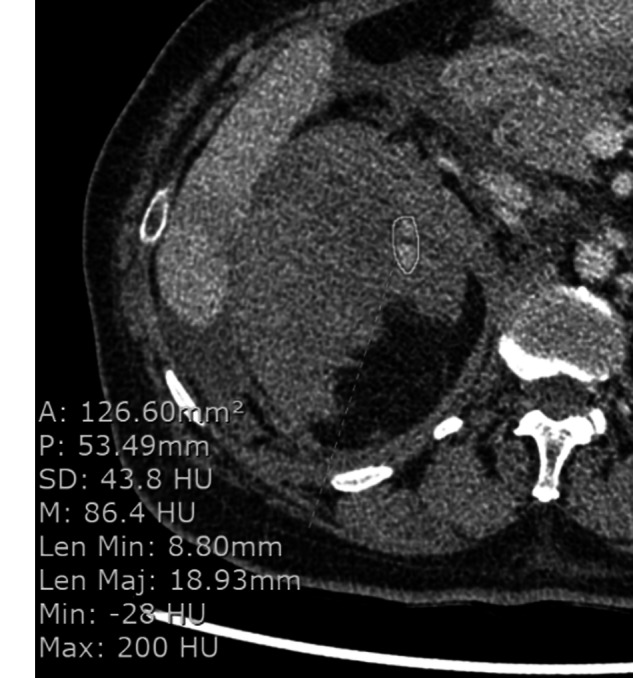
Example of measurements of active vascular contrast extravasation on a CT image using electronic calipers. A area (mm^2^), Len Max maximum length (mm), Len Min minimum length (mm), M mean (Hounsfield units; HU), Max maximum (HU), Min minimum (HU), P perimeter (mm), SD standard deviation (HU)
